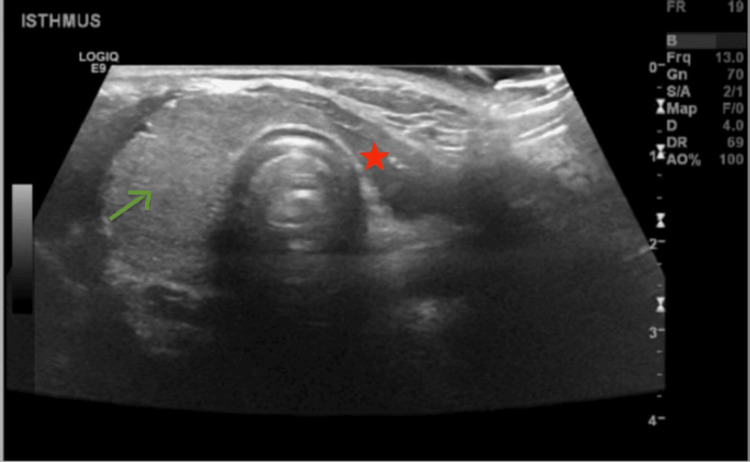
Ultrasound image showing absence of the left thyroid lobe and compensatory hypertrophy of the right lobe in a 15-year-old female with thyroid hemiagenesis. Thyroid ultrasound (longitudinal section of anterior neck) showing midline-located thyroid gland demonstrating absence of the left thyroid lobe and compensatory hypertrophy of the right lobe in a 15-year-old female with thyroid hemiagenesis. The isthmus was present and measured 0.27 cm. A scale bar is included. Green arrow: enlarged right lobe.
